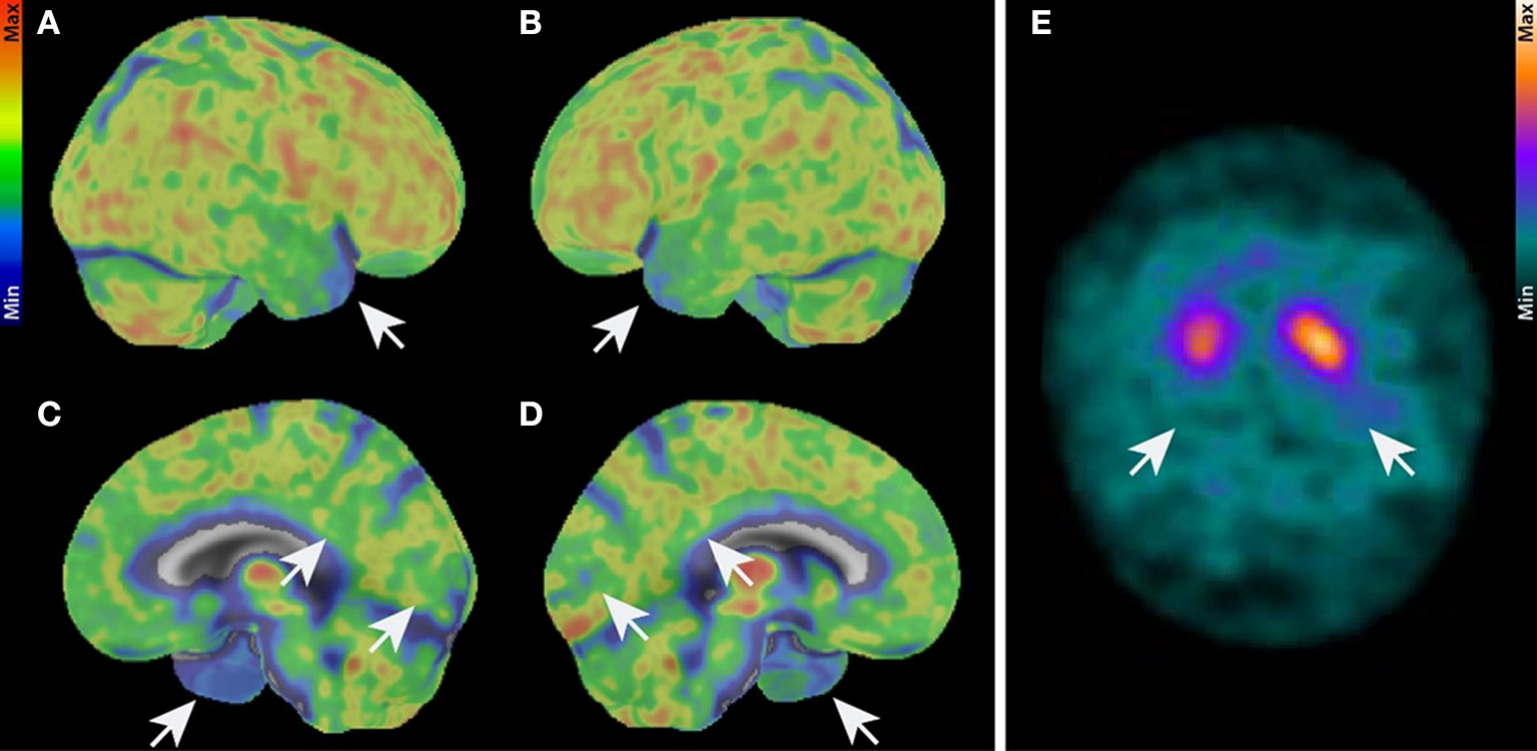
Imaging. (A–D) 18F-FDG-PET. Brain hemisphere surface projections of ^18^F-FDG- uptake in right lateral (A), left lateral (B), right medial (C), and left medial (B) views show reduced tracer uptake in the temporal cortex, the posterior cingulate, precuneus, and slightly reduced uptake in the visual cortex (white arrows). (E)
